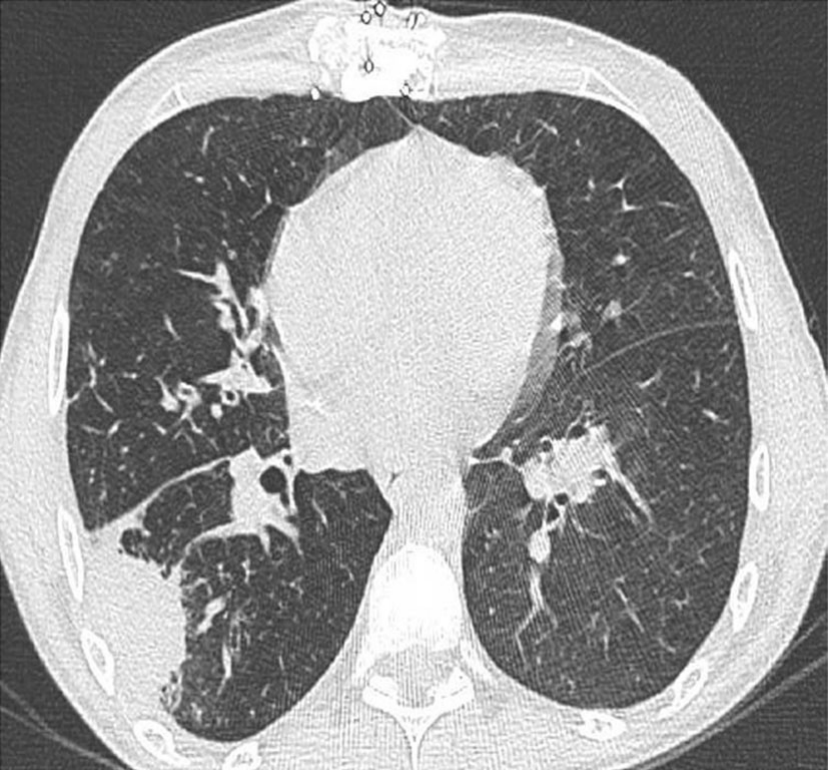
HR CT chest scan demonstrating a biopsy-proven *Aspergillus sp.* infection. The pleural-based lesion shows surrounding glass ground appearance on the free edge. The presence of this intrapleural lesion shows the halo sign, a lesion typically seen early in Aspergillosis
